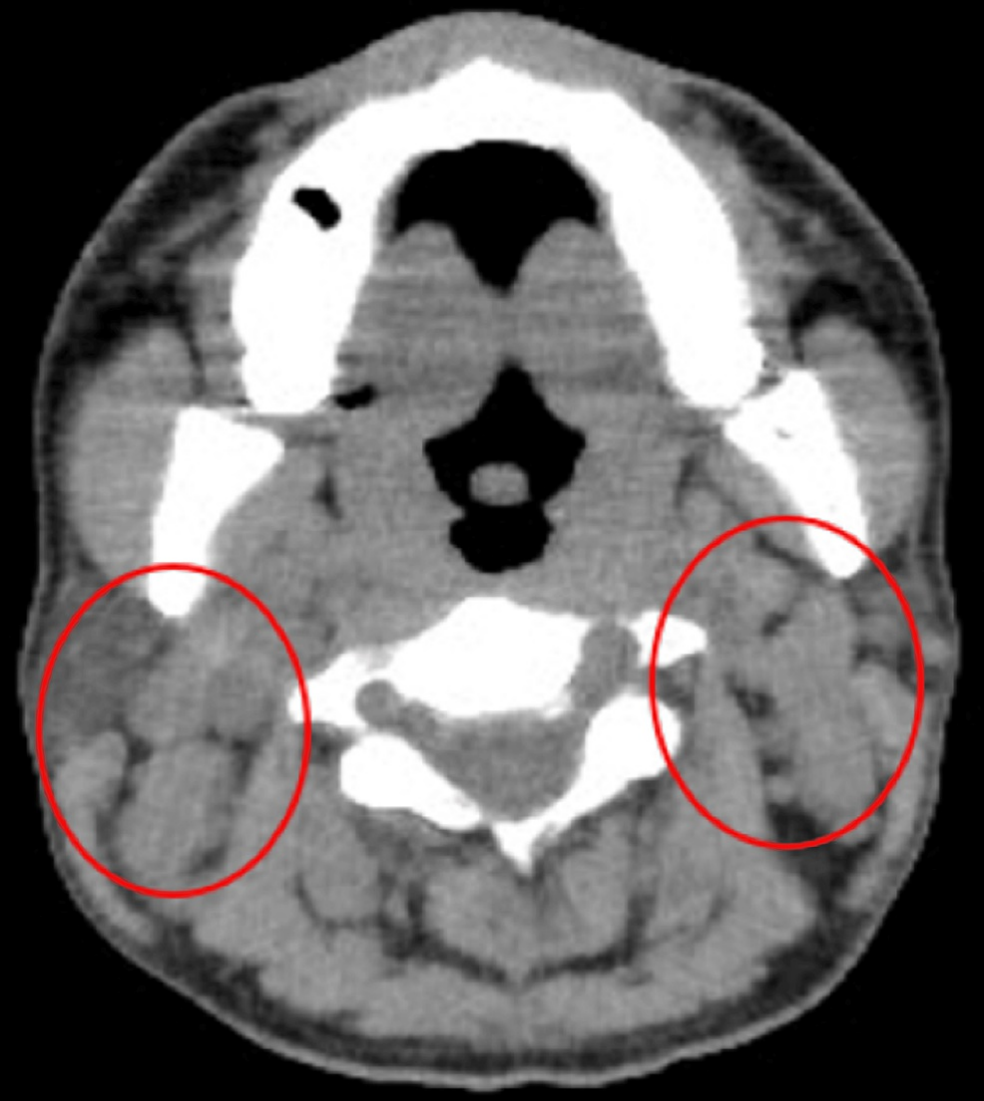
Computed tomography revealed multiple enlarged bilateral cervical lymph nodes (red circles)
